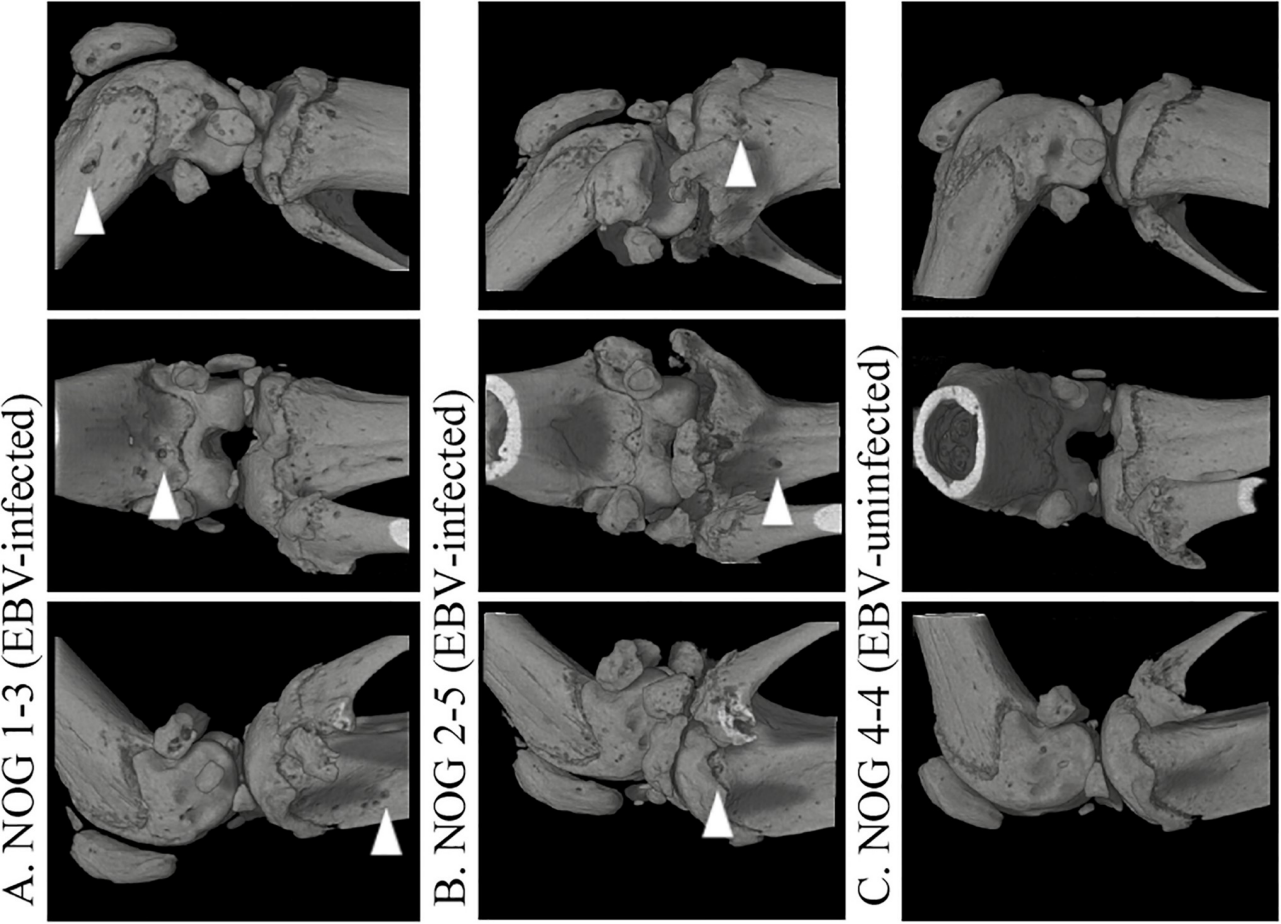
3D-CT images of knee joints in EBV-infected and -uninfected hu-NOG
